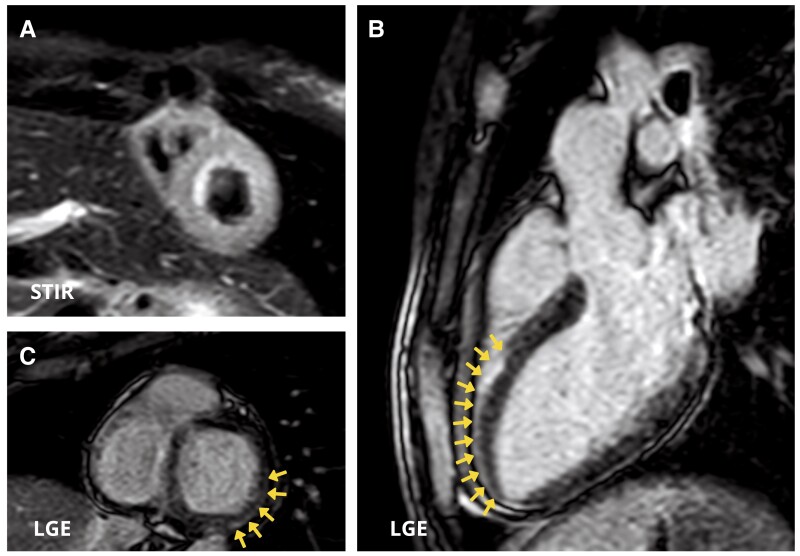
Cardiac magnetic resonance images. (*A*) Short-axis view showing diffuse oedema in the left ventricular wall in T2 Short Time inversion recovery (STIR) sequences. (*B* and *C*) Three-chamber view (*B*) and short-axis view (*B*) demonstrate myocardial late gadolinium enhancement with sub-epicardial distribution in the mid-apical segment of antero-septal wall and in the basal segment of inferior and infero-lateral walls (arrows).
